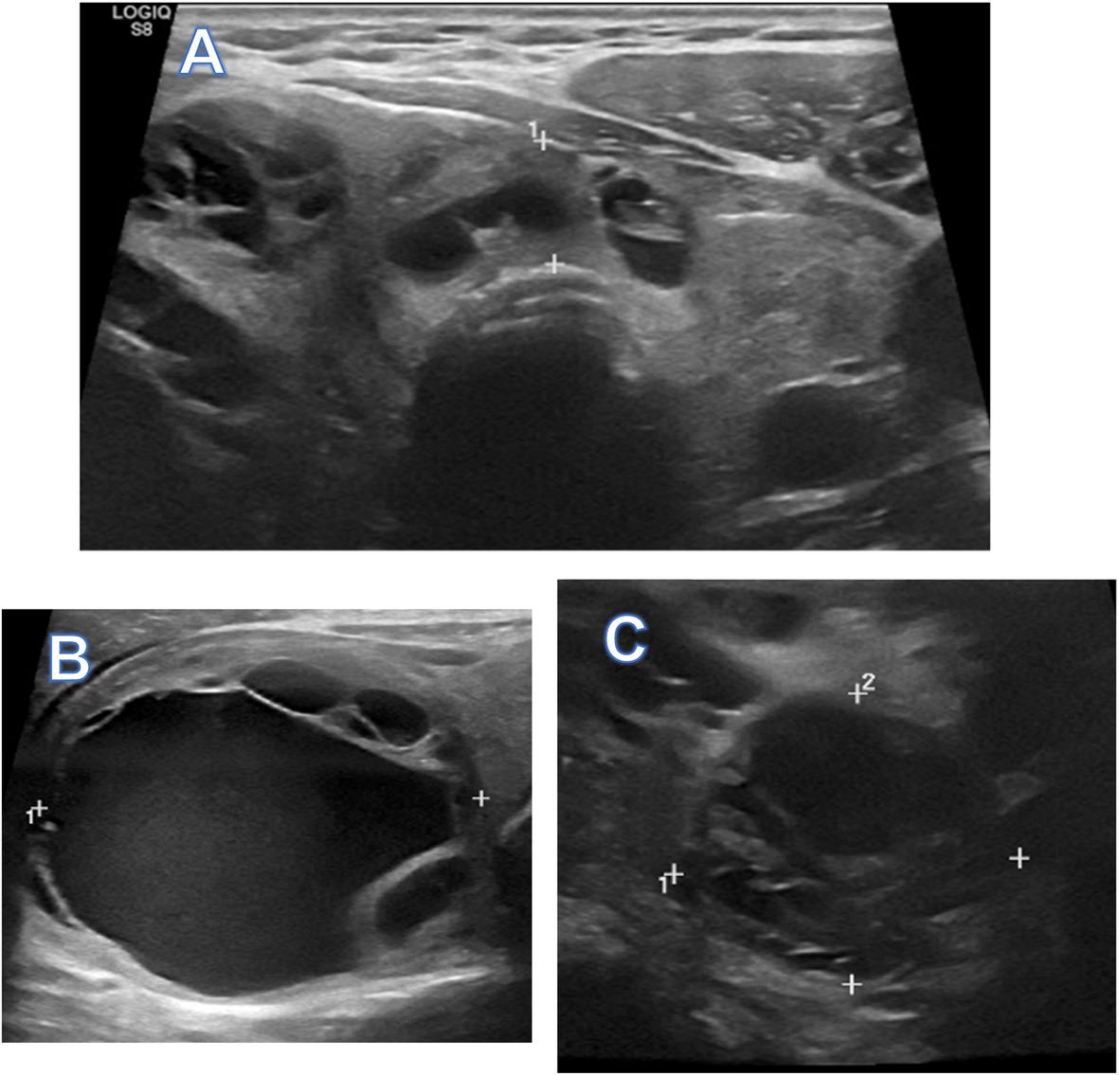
Transverse view ultrasound. A Asymmetrically enlarged, heterogenous thyroid. B Right mid thyroid nodule with mixed cystic and solid components measured 3.9 × 4.3 × 4.9 cm in size. C Right inferior thyroid nodule with mixed cystic and solid components measured 2.5 × 2.2 × 2.9 cm in size
